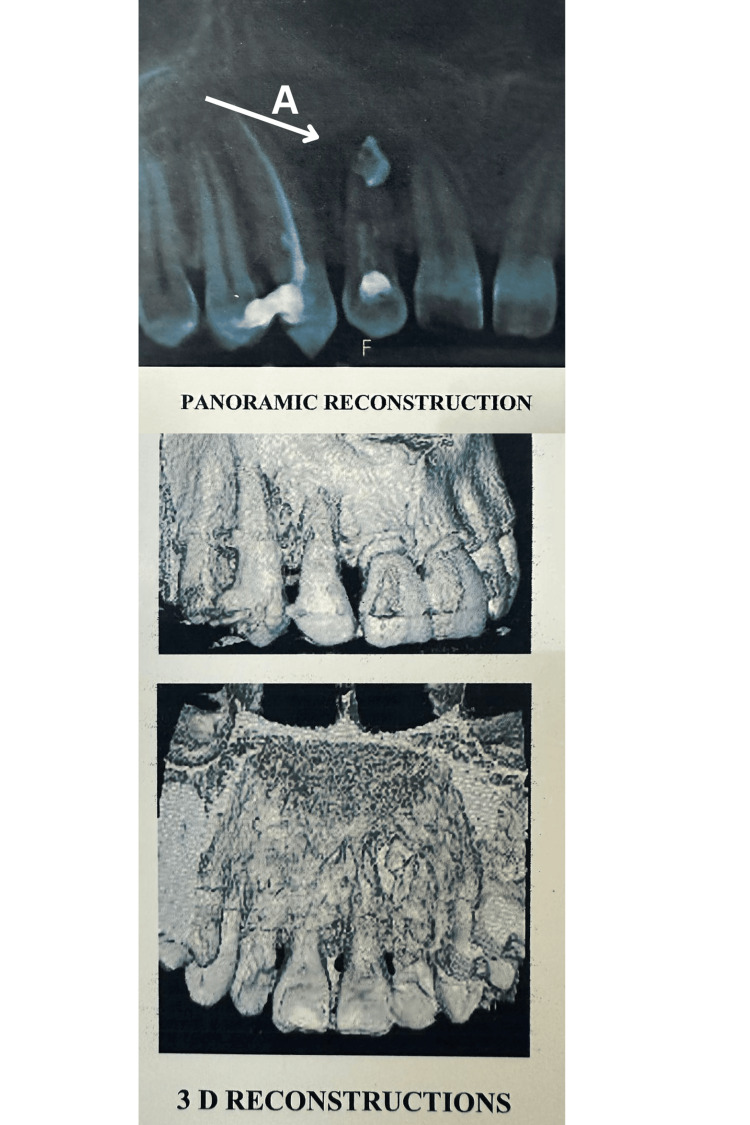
Panoramic reconstruction view and three-dimensional view of CBCT i.r.t 12 A: Periapical Radiolucency
